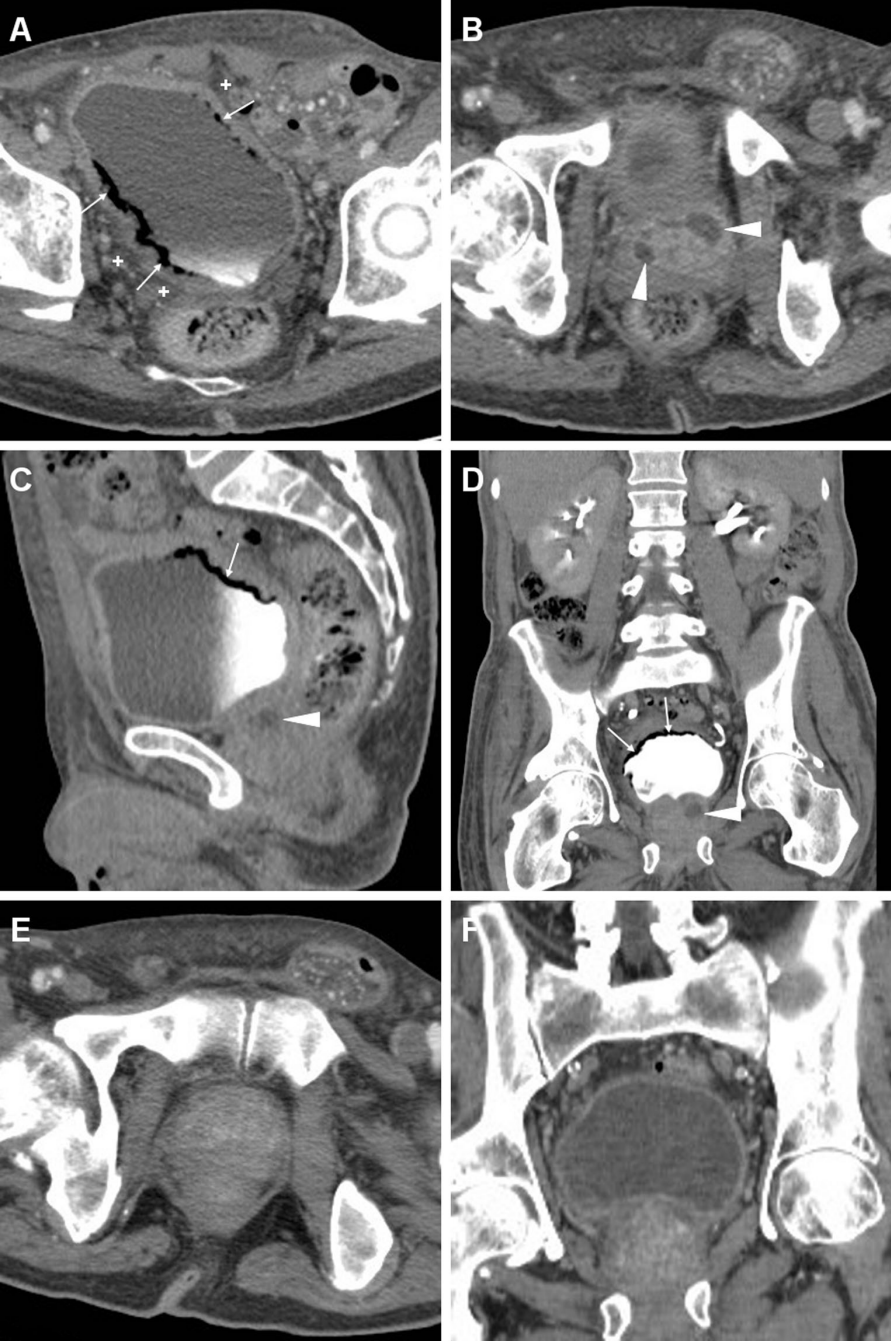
Emphysematous cystitis in a 69-year-old man with congestive heart failure, diabetes and chronic obstructive lung disease, suffering from urinary frequency and pain. CT urography (a–d) revealed distended urinary bladder with linear gas-attenuation changes (*thin arrows*) along the right lateral and upper posterior walls. Associated findings included inflammatory stranding (+) of the perivesical fat planes and a small bilobated fluid-like intraprostatic collection consistent with abscess (*arrowheads*). Follow-up CT (e, f) 3 months later revealed resolution of changes after prolonged antibiotic therapy
